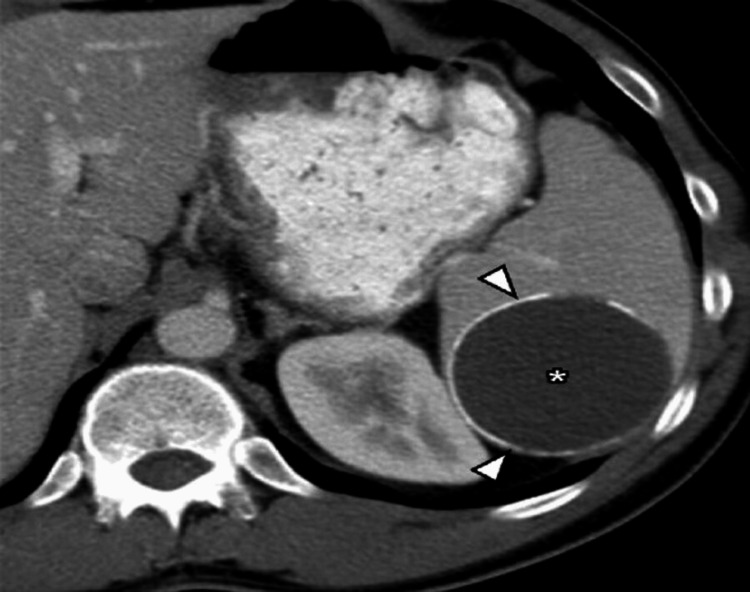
Splenic pseudocyst. Contrast-enhanced CT shows a well-defined cyst in the spleen (*) with a peripheral rim of calcification (arrowheads) and no internal septations, presumed to be a post-traumatic pseudocyst. Used with permission from Kaza et al. [4]
